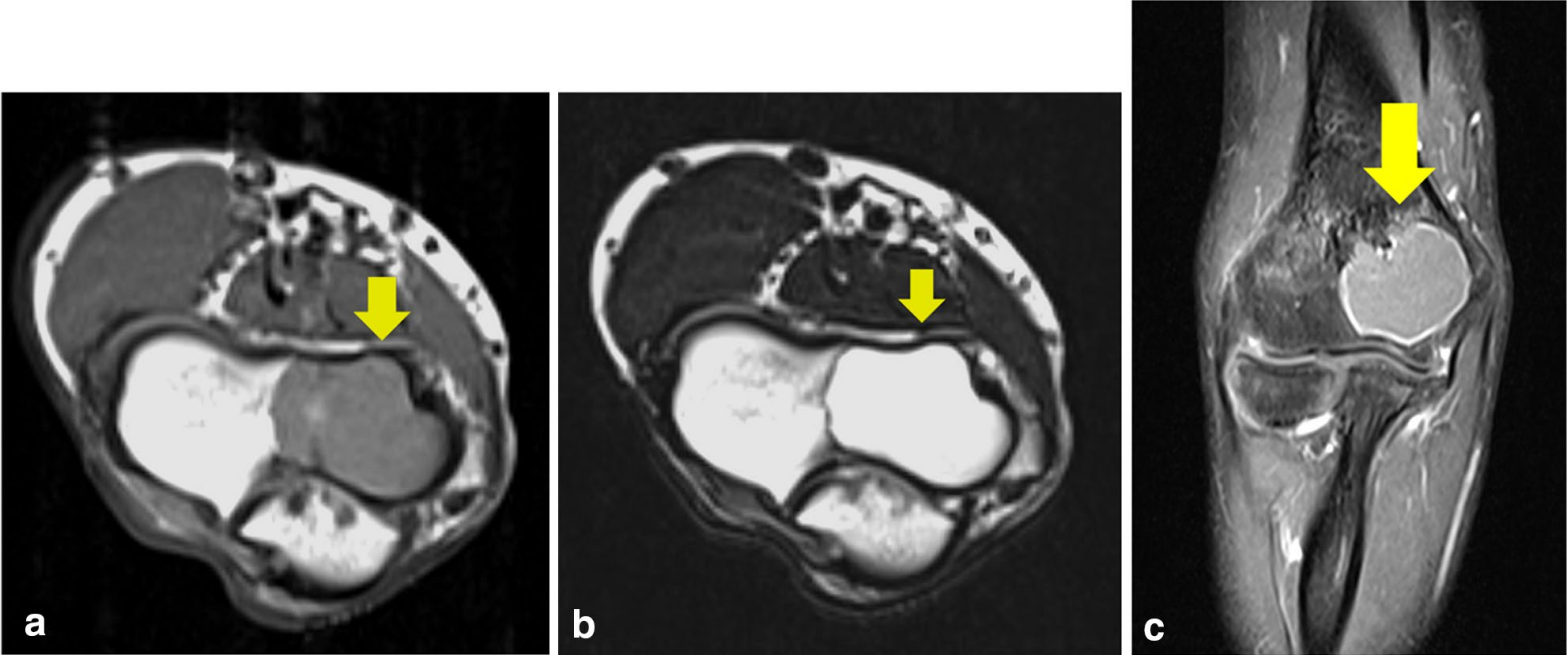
Follow-up magnetic resonance imaging (MRI) findings. The lesion appears hypointense to muscle on T1-weighted MRI (a), and predominantly hyperintense on T2-weighted fat-saturated sequences (b). Post-gadolinium sequences show peripheral enhancement of the cyst (c). MRI reveals simple bone cyst or aneurysmal bone cyst in the distal humerus (*yellow arrow*).
